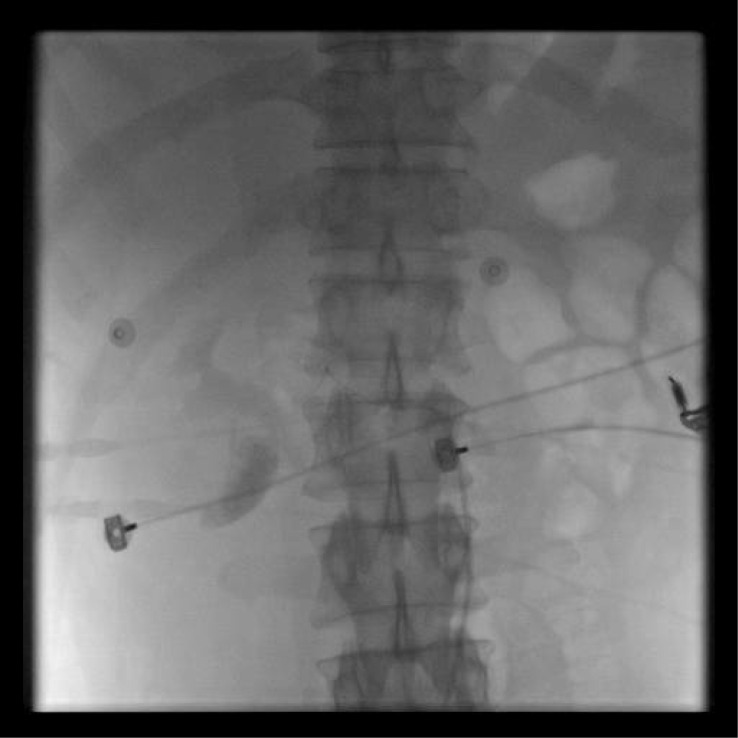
Abdominal plain radiograph revealing a right renal arteriovenous fistula.
